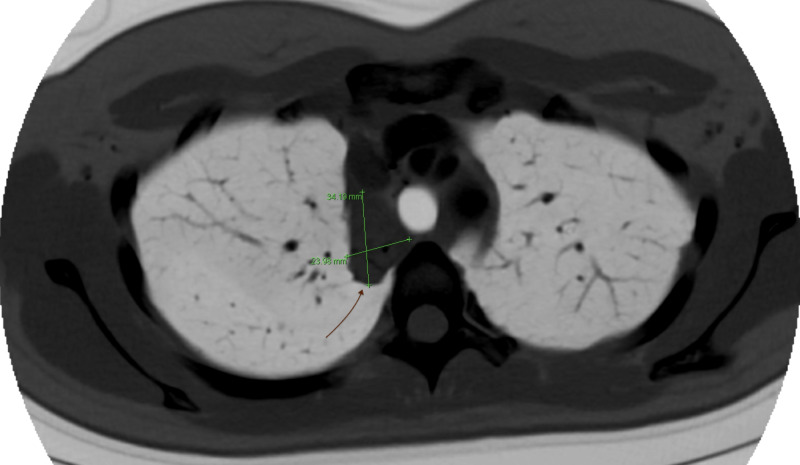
Chest CT With Contrast A high right paratracheal and medial right apical heterogeneous soft tissue with central areas of calcification.
